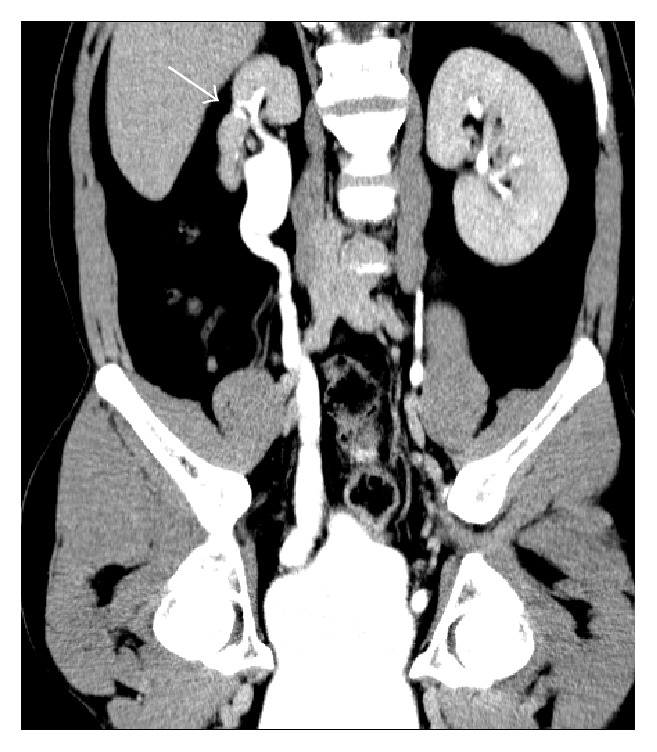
Postpyelonephritic scar. Coronal reformatted contrast-enhanced CT (excretory phase) shows a focal postpyelonephritic scar (arrow) in the upper-third of the right kidney with dilatation of ipsilateral renal pelvis and ureter.
